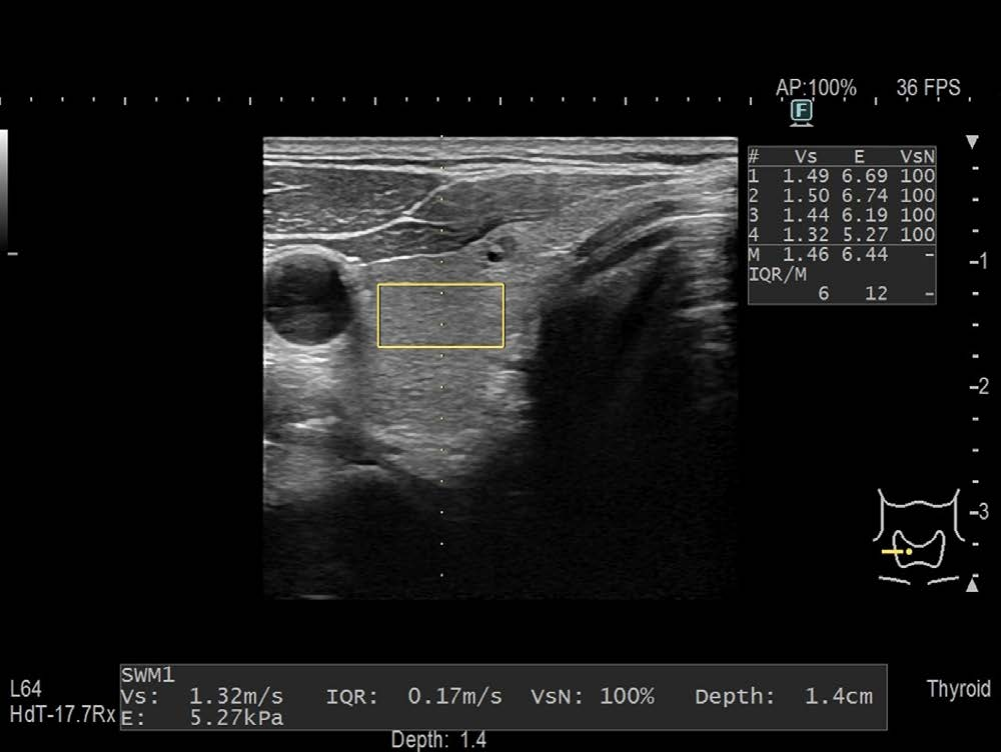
Identification of thyroid stiffness using shear wave elastography. Thyroid stiffness was determined using elastography. The shear wave velocity of the thyroid was measured using B-mode ultrasonography. A target region was identified within a region of interest as shown in the rectangle.
